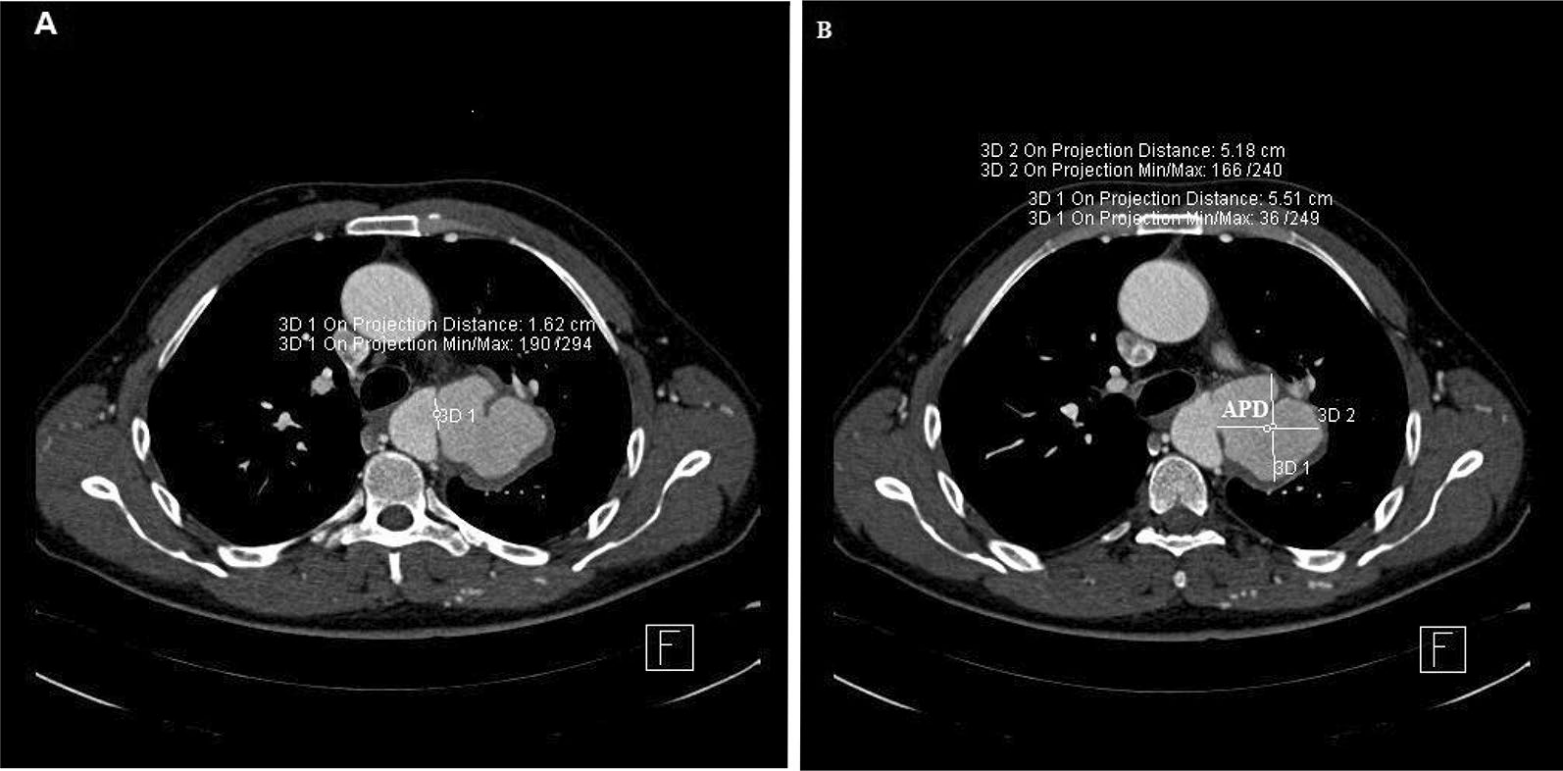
2D computed tomography angiography scan, axial views, shows the 52 × 55 mm pseudo-aneurysm with a small neck communicated to the aortic lumen (*APD* aortic pseudo-aneurysm)
